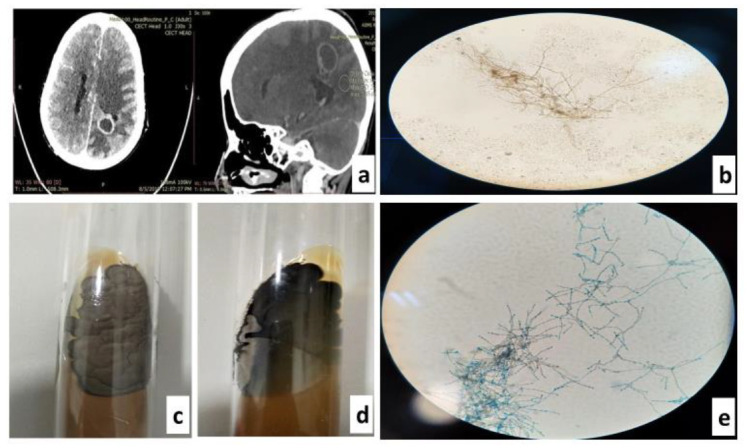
a. Contrast enhanced Magnetic resonance imaging scan revealing a large peripherally enhancing well defined cystic space-occupying axial lesion in left parietal cerebral lobe, with mass effect. b. Pus sample showed numerous pigmented septate hyphae on potassium hydroxide (KOH) mount (Magnification:400x). c. Culture tube showing olivaceous black colonies on obverse. d. Culture tube showing black non-diffusible pigment onreverse. e. Slide culture showing chains of brown, smooth-walled, single-celled, ellipsoid conidia on Lactophenol cotton blue mount (Magnification:400 x).
